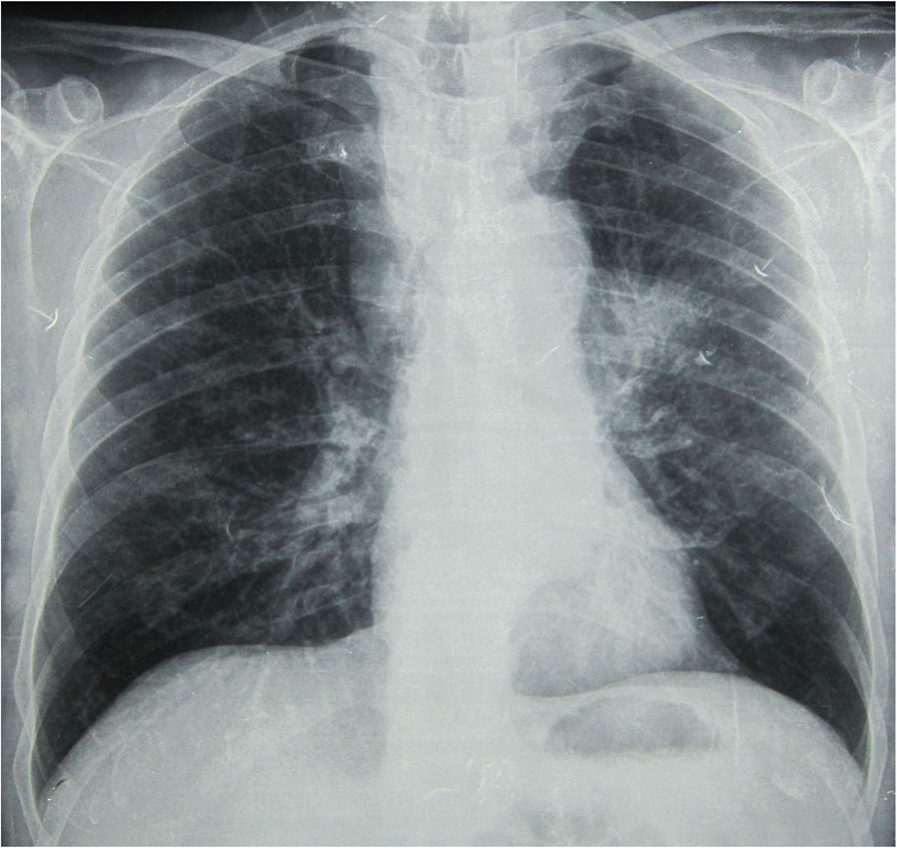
Chest x-ray on presentation, showing mediastinal widening and left parahilar infiltrate
